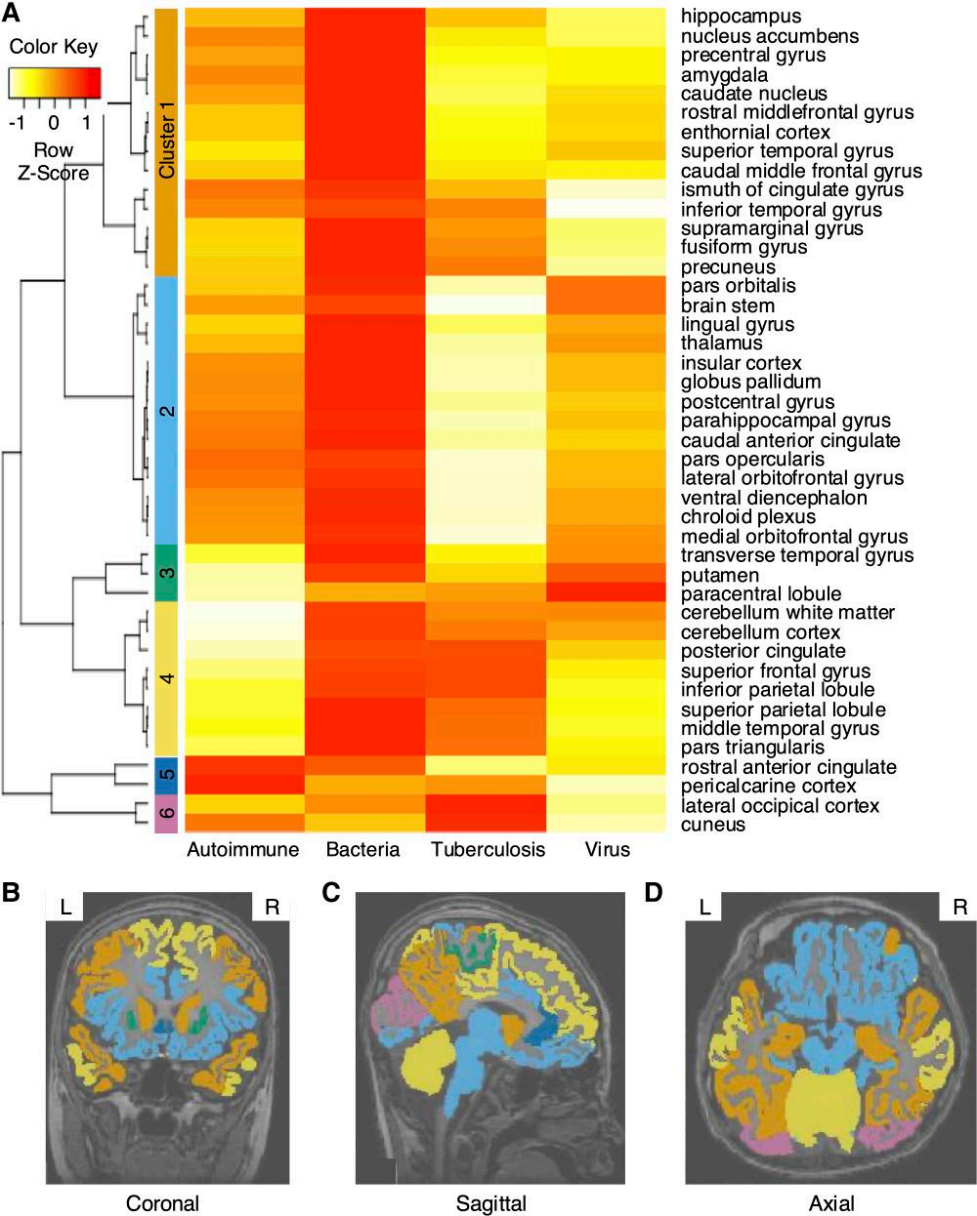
Hierarchical clustering results based on predictive performance of unimodal models using brain MRI. (A) The 43 brain regions were divided into 6 clusters. (B–D) Visualization of hierarchical clustering of each brain region overlaid on brain MRI images. (B) Coronal, (C) sagittal and (D) axial section. Hierarchical clustering was performed using an agglomerative approach with average linkage and Euclidean distance as the dissimilarity metric. Clustering was conducted on an internal dataset derived from the results of the MRI unimodal model. The dataset included autoimmune (*n* = 45), bacterial (*n* = 46), tuberculosis (*n* = 30) and viral (*n* = 170) cases.
